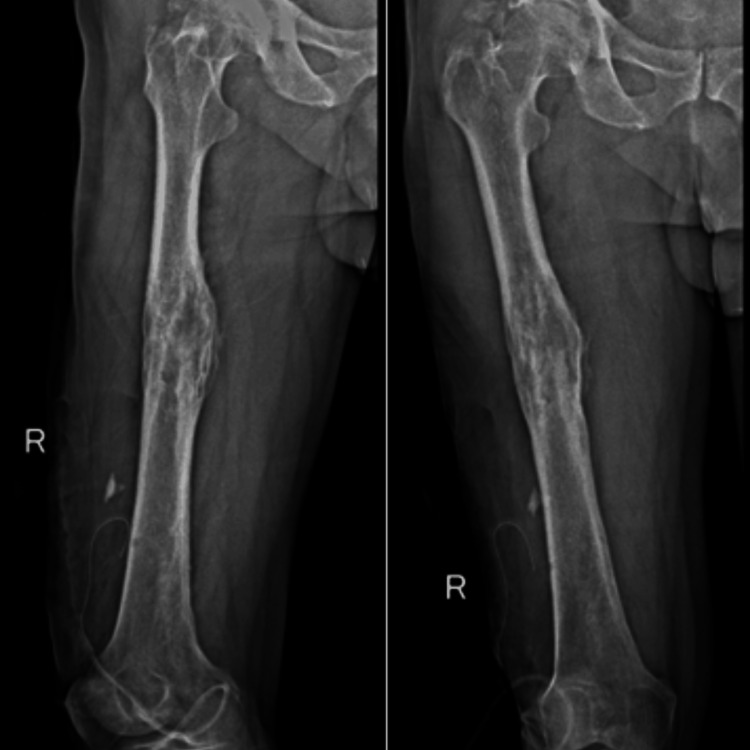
Postoperative radiographs of the right femur show the intraoperatively inserted drain.
